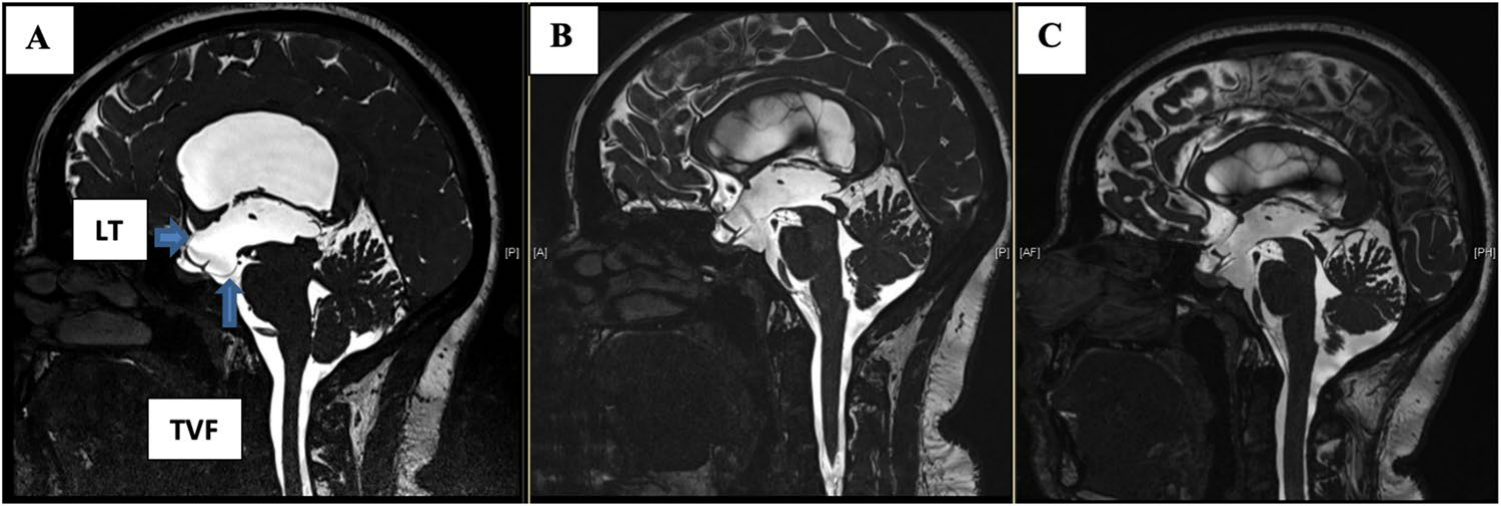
Pre-, postoperative and 3-months mid-sagittal CISS* magnetic resonance images of a 27 year old patient, who showed a preoperative Heidelberg score of + 2 (TVF + 1 and LT* + 1) (A), postoperatively, the TVF and LT went to a grade of 0 each, thus the postoperative Heidelberg score was 0, as seen in the middle image (B). After three months, the LT showed a reduction such that the Heidelberg score decreased to -1 as shown in the right image (C)
